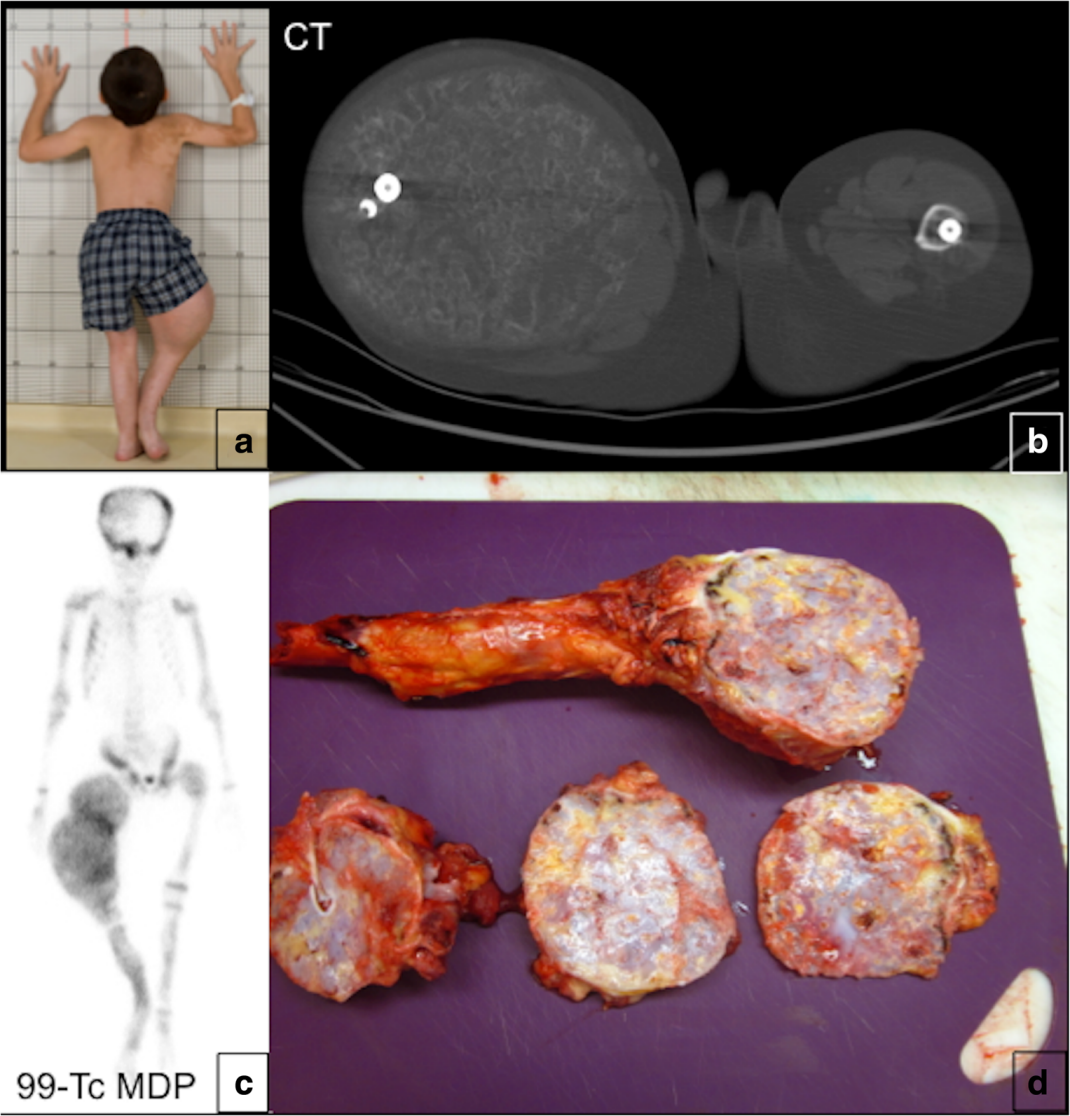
Benign myxoid bone matrix transformation in fibrous dysplasia (FD). a A patient with known FD of the femurs presents with an enlarging right thigh mass. b Axial unenhanced CT of the lower extremities shows a large heterogeneous mass in the right thigh replacing femur and causing mass effect on thigh muscles. Please note a normal position of the intramedullary road in the left femur. c The mass in the right thigh shows a focal 99m-Tc MDP radiotracer uptake. d Subsequently, the patient developed the same complication in the left leg. The image demonstrates extensive myxoid degeneration of the left femur
